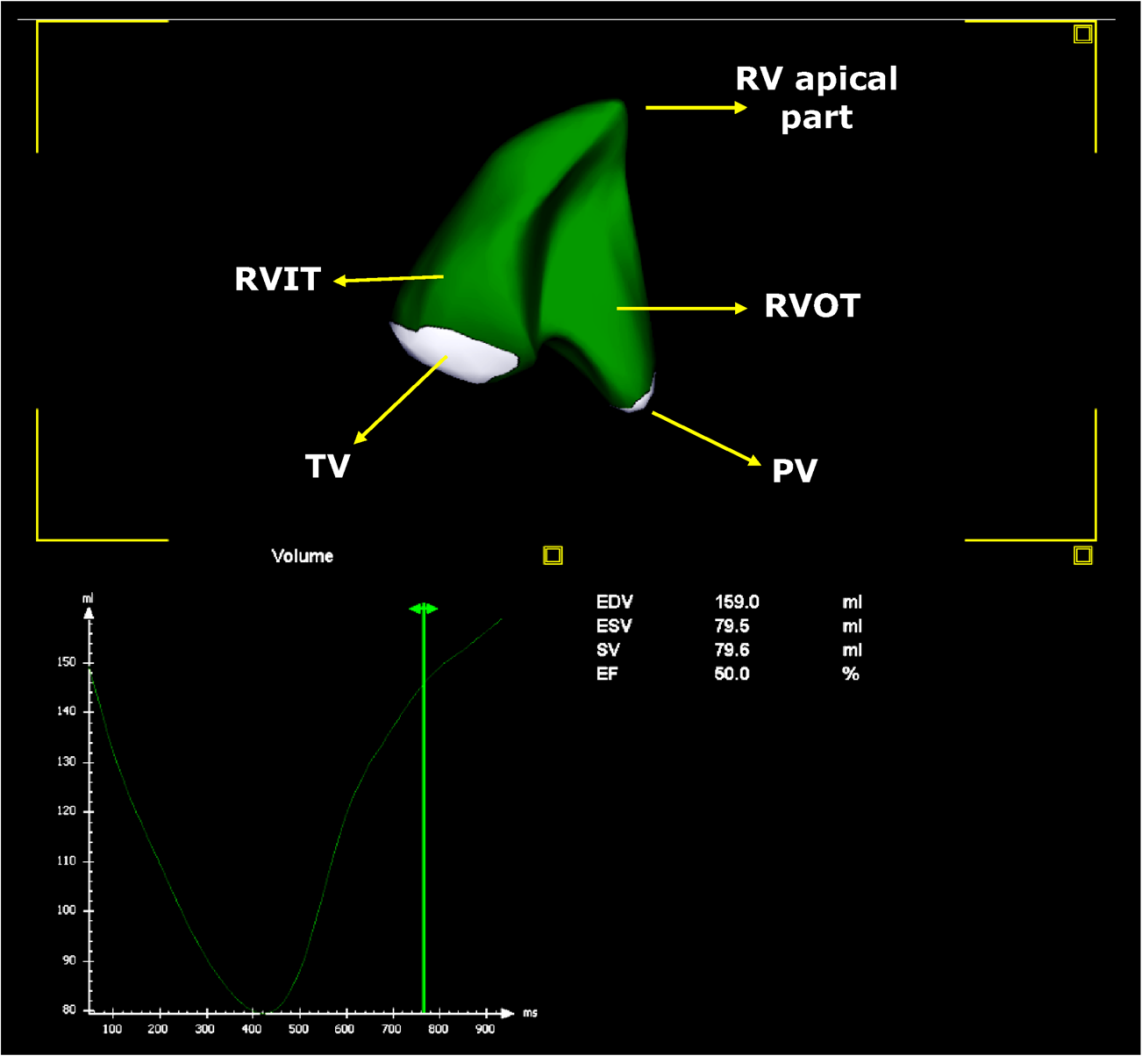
Right ventricular assessment by 3D-echocardiography. This picture shows a 3D-echocardiographic reconstruction of the right ventricle (RV). The software provides a motion picture of the RV as well as the volume-time curve and RV measures, such as RV end-diastolic volume (EDV), RV end-systolic volume (ESV), RV stroke volume (SV), and RV ejection fraction (EF)
